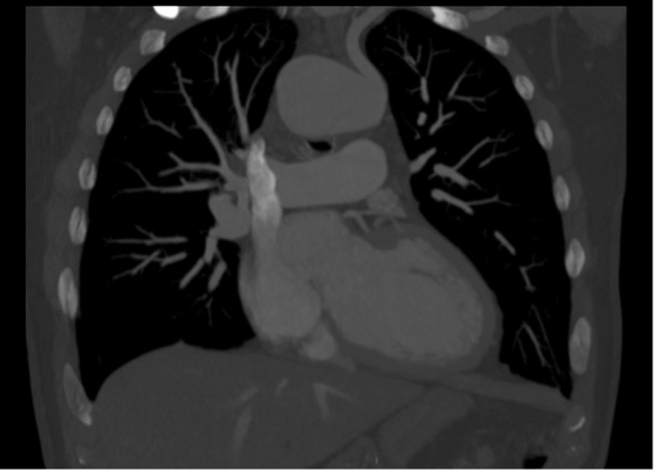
CT imaging demonstrating the anatomical relationship between the diverticulum and airway.
